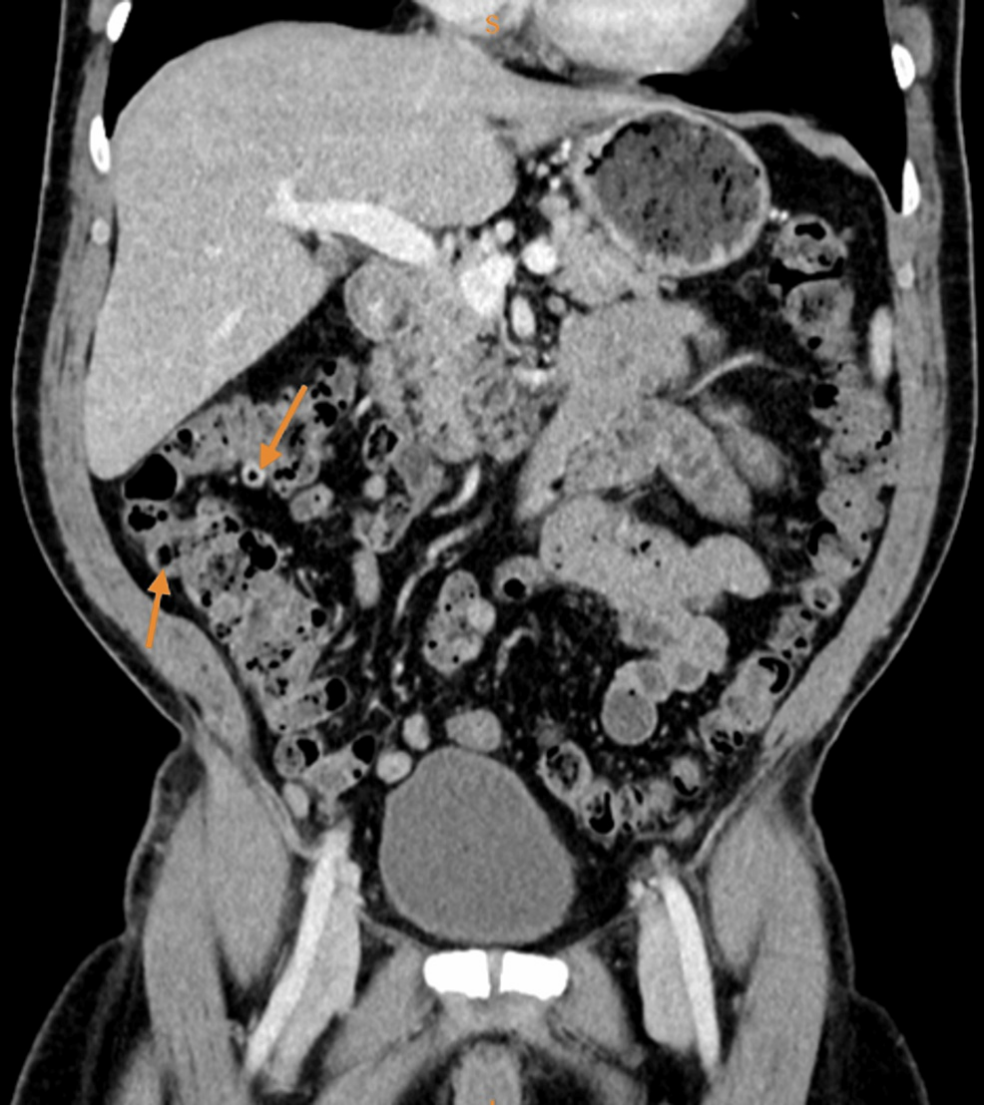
CT coronal scan demonstrating right-sided diverticulitis.
